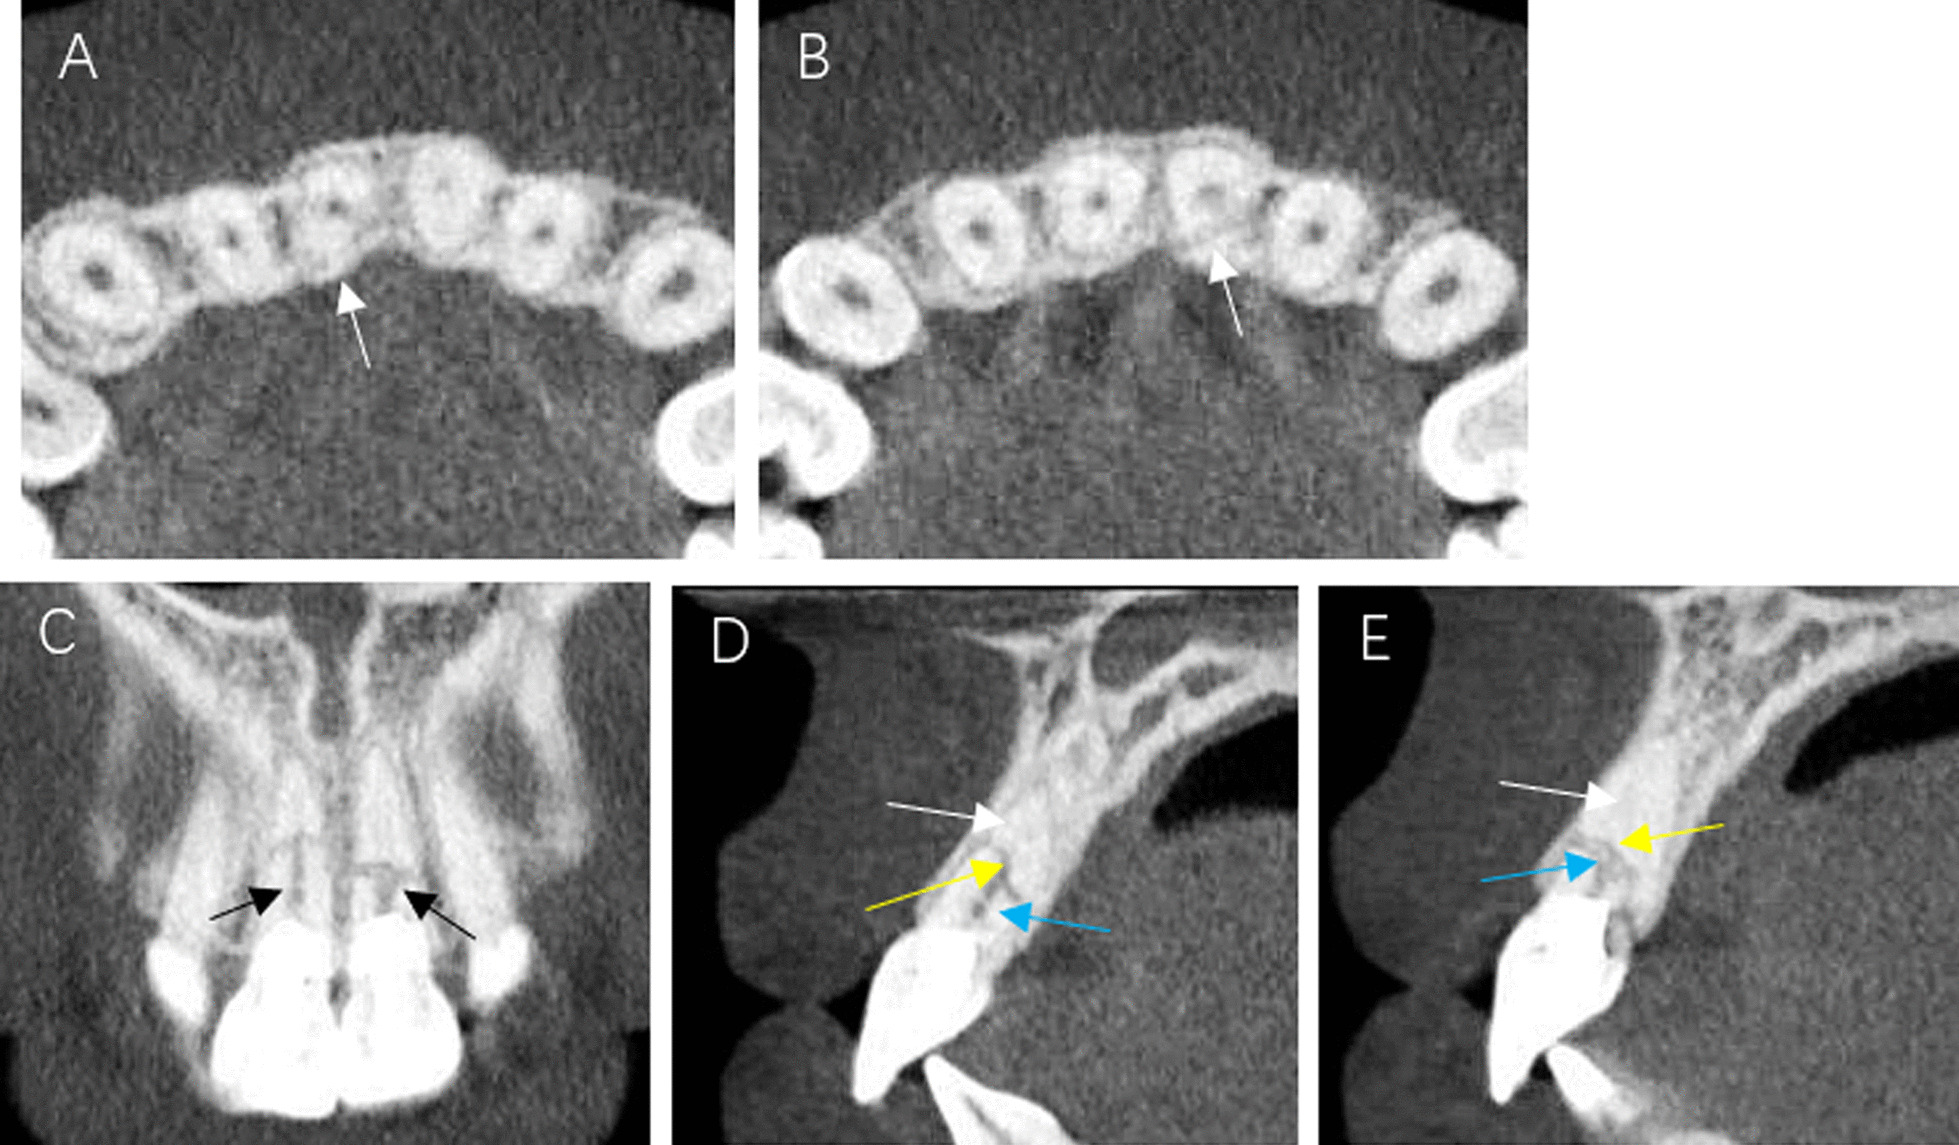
CBCT imaging of the root-fractured teeth taken at the 36-month review. A Axial review of tooth #11 B Axial review of tooth #21 C Coronal view Intracanal calcification in the coronal fragment is evident (black arrow) D Saggital view of tooth #11. Canal obliteration in the apical fragment is evident (white arrow) as is calcific tissue between the fractured fragments (yellow arrow). There is also mineralized tissue evident is the coronal fragment (blue arrow). E Saggital view of tooth #21 showing a similar calcific response to tooth #11
